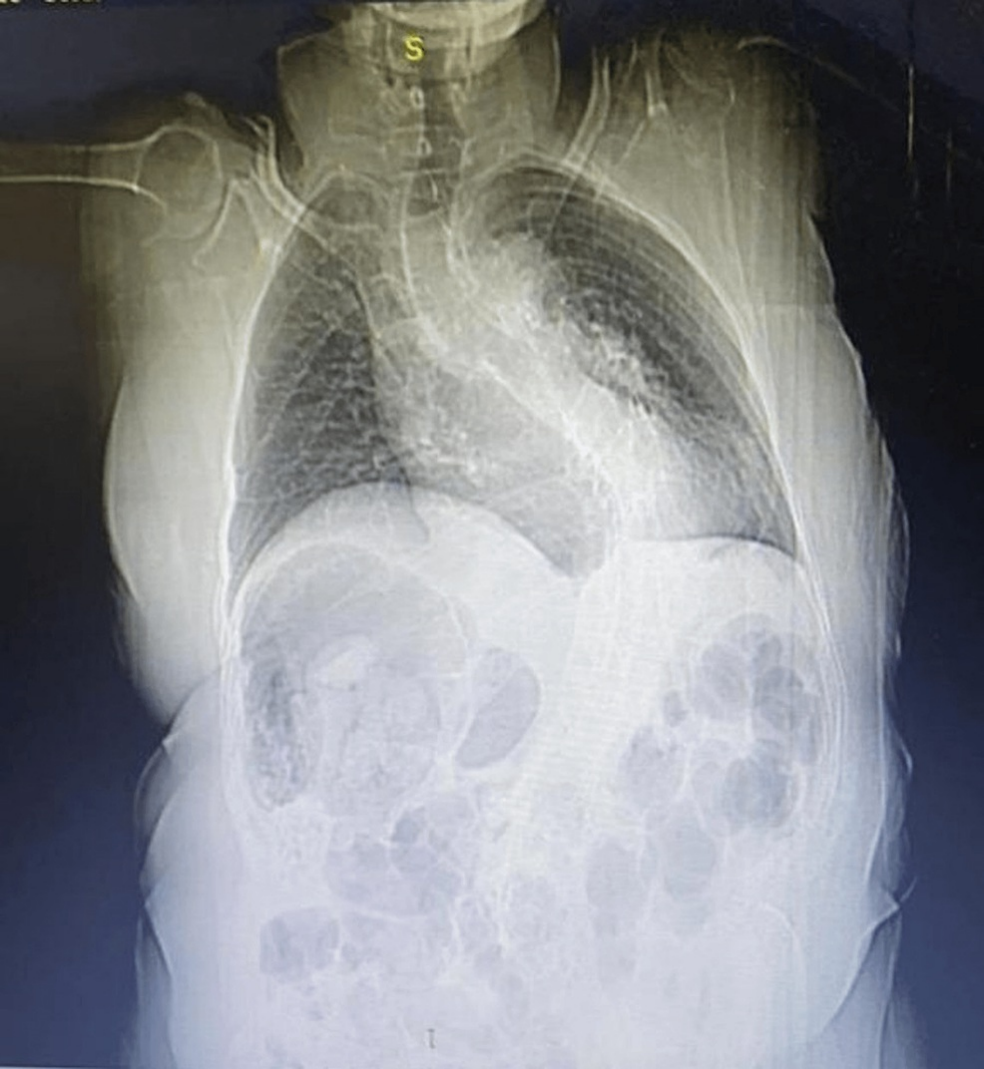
Chest radiograph (CXR) showing severe scoliosis (Cobb angle of ≥50)
